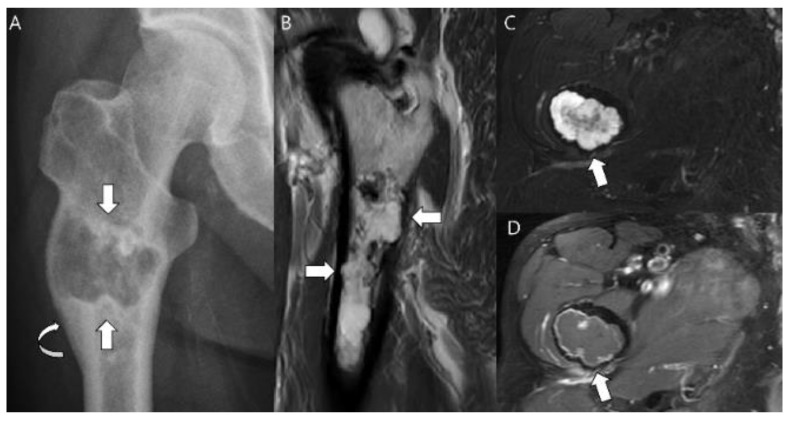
Chondrosarcoma grade 2 of the proximal femur in a 71-year-old man. (A) Anteroposterior radiograph reveals a lytic lesion in the proximal femur (arrows) resulting in cortical thickening and periosteal reaction (curved arrow). (B) Sagittal T2-weighted image shows a markedly high-signal lesion with deep endosteal scalloping (arrows). (C) Axial T2-weighted image with fat suppression and (D) axial T1-weighted enhanced image demonstrates a lobulated chondroid tumor with focal bone expansion (arrows).
